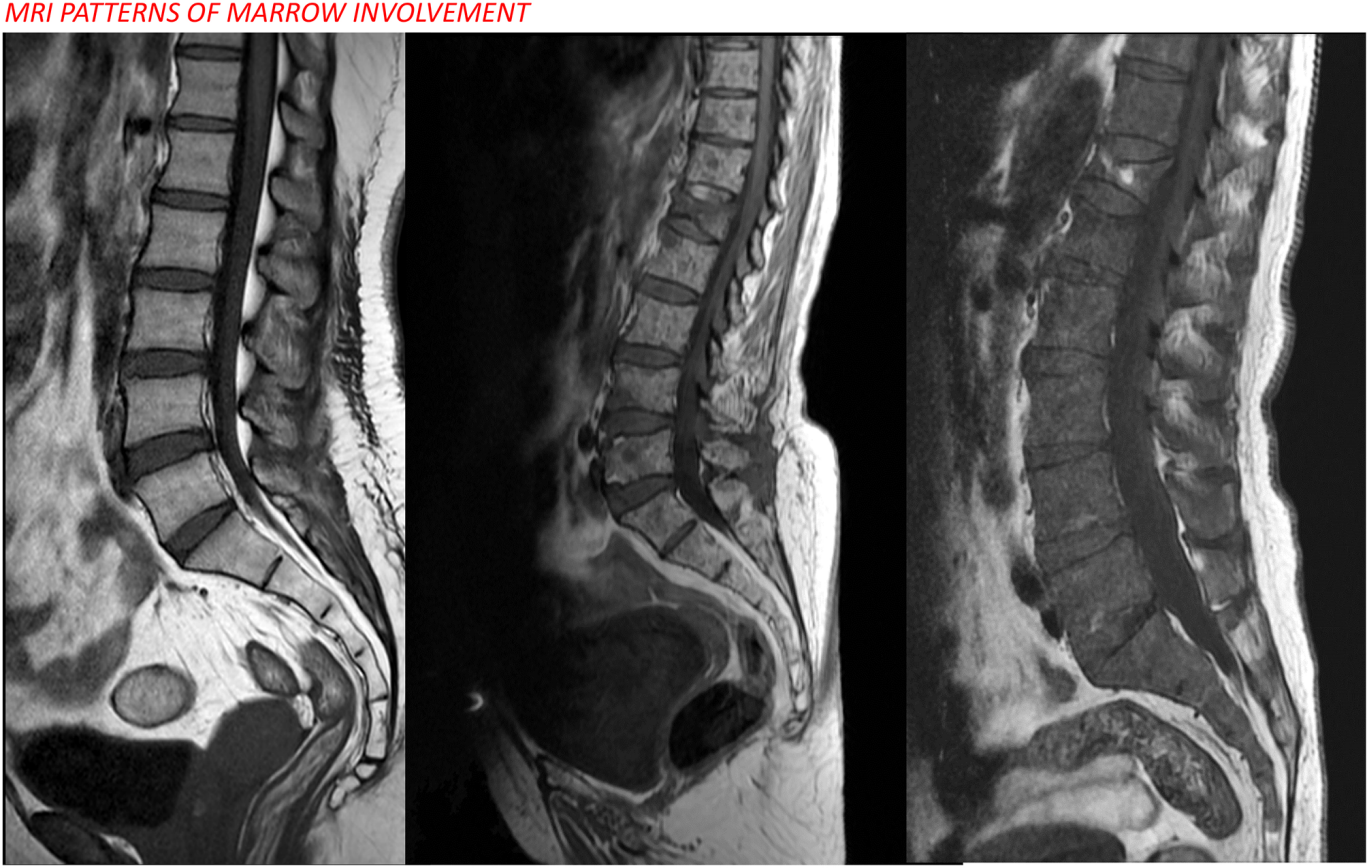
MRI patterns of marrow involvement in multiple myeloma showing normal, focal, and diffuse patterns from left to right.
